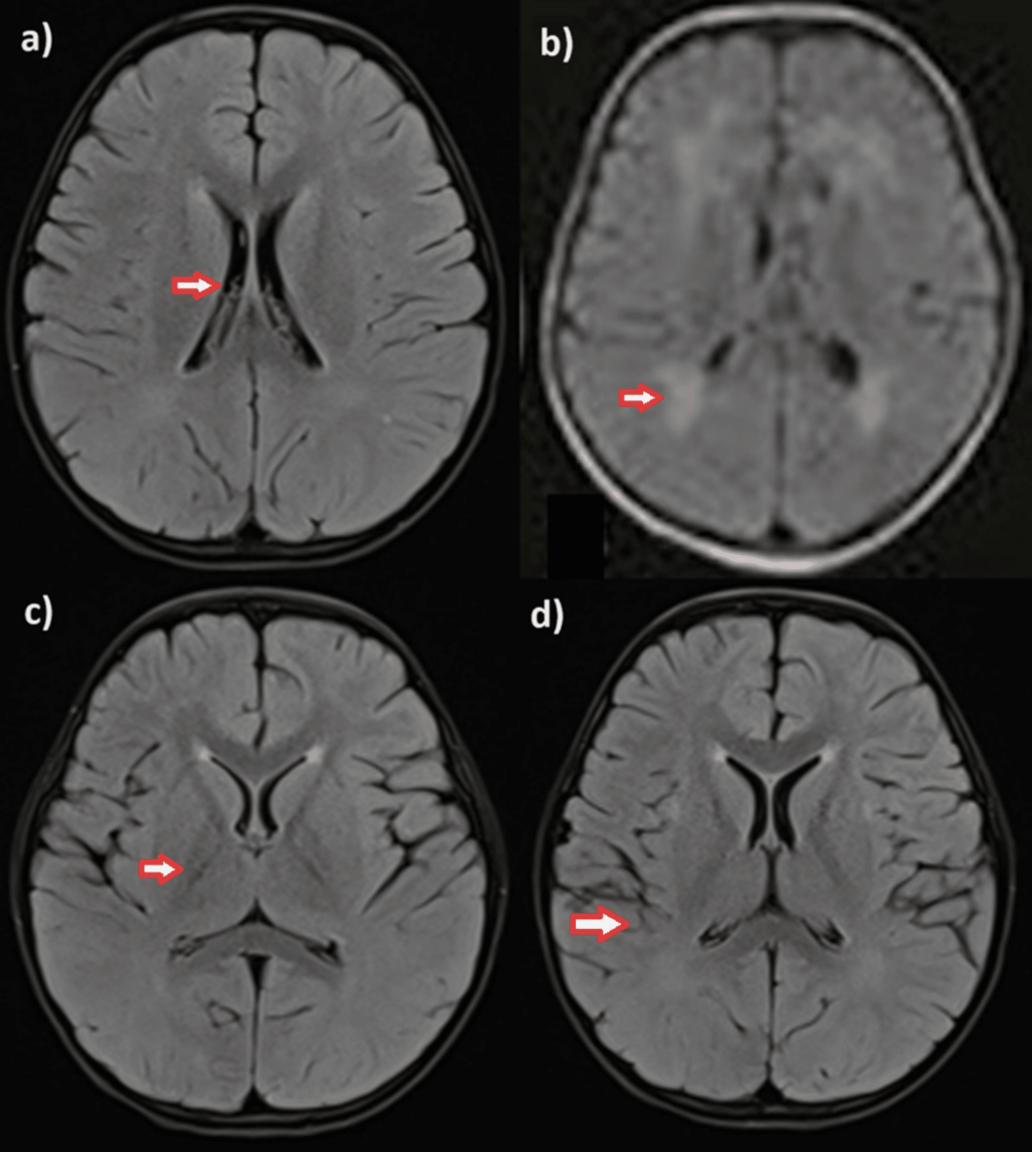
MRI images fluid-attenuated inversion recovery sequence. (a) Mild enlargement of lateral ventricles. (b) Hyperintensity in bilateral periventricular area. (c) subtle hyperintensity in the putamen area. (d) subcortical area involvement.
